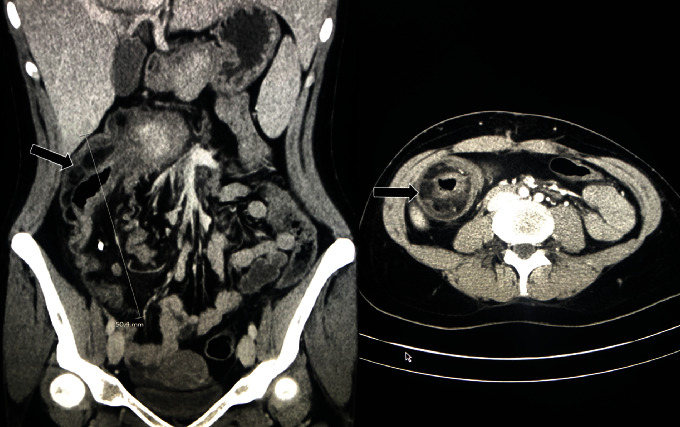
Abdominal computed tomography showing the ileocecal intussusception (left: coronal; right: transverse).
